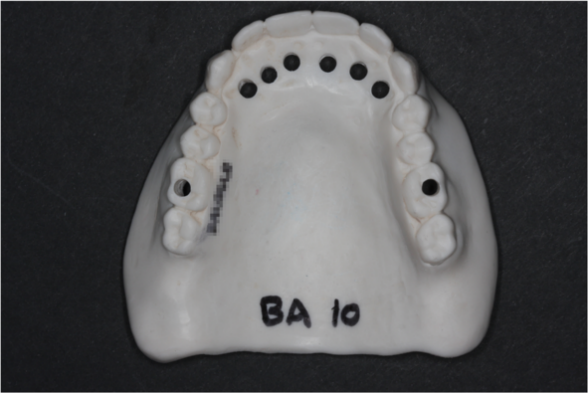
Radiographic guide with pits next to extraction sites
